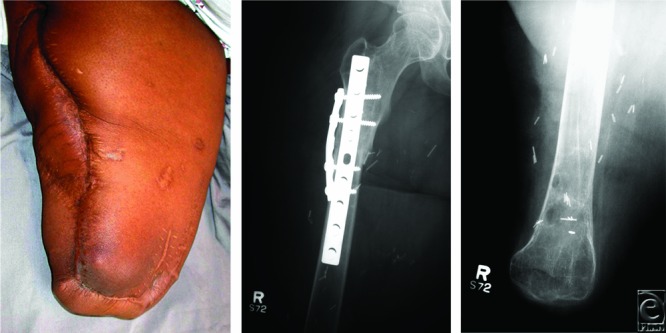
Thirty months after surgery. There has been excellent healing of the right thigh with a functional hip joint (*left*). Anteroposterior radiographs show osteosynthesis of the distal tibia and proximal femur (*middle*) and the proximal tibia serving as the distal tip of the rotationplasty (*right*).
